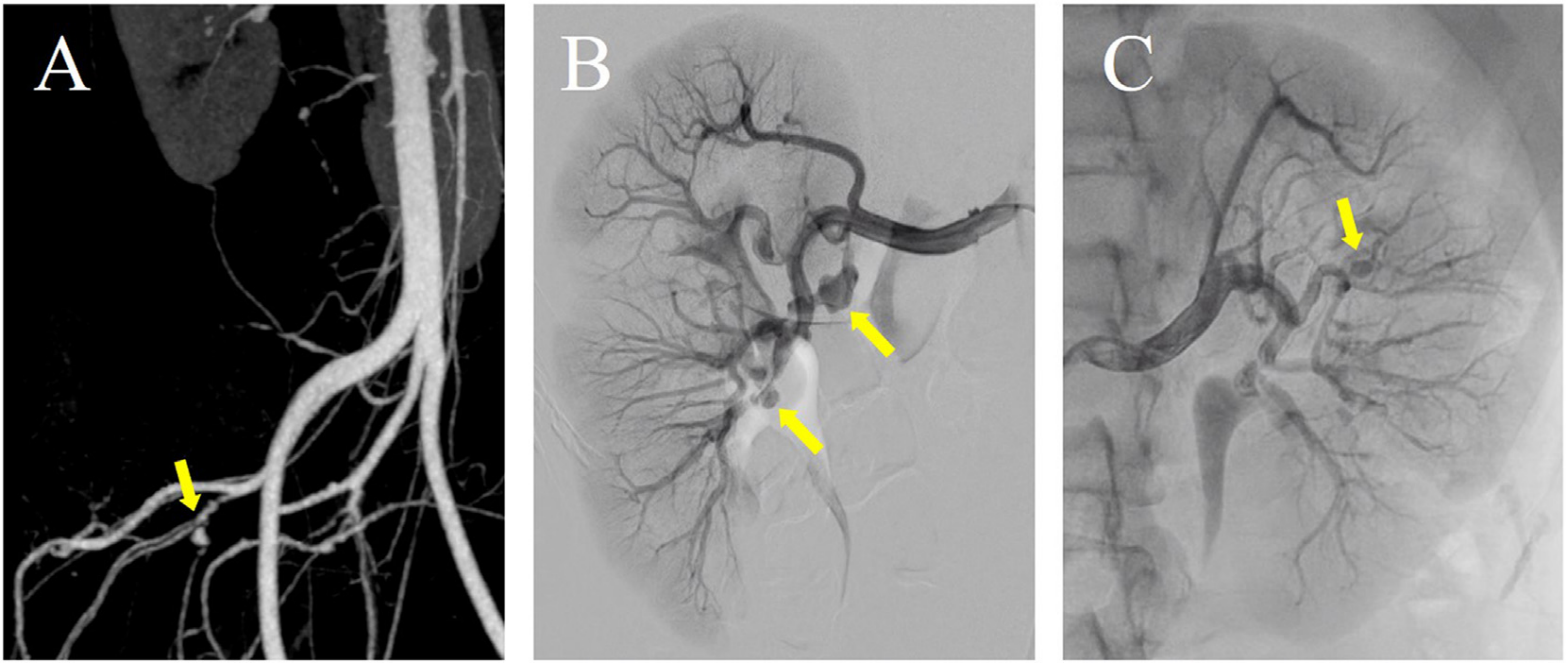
Computed tomography angiography, showing a focal stenosis in the left internal iliac artery (arrow) (A). Selective renal angiography, showing multiple stenosis and post stenotic dilatations (arrows) at distal sites of the right (B) and left (C) renal arteries.
